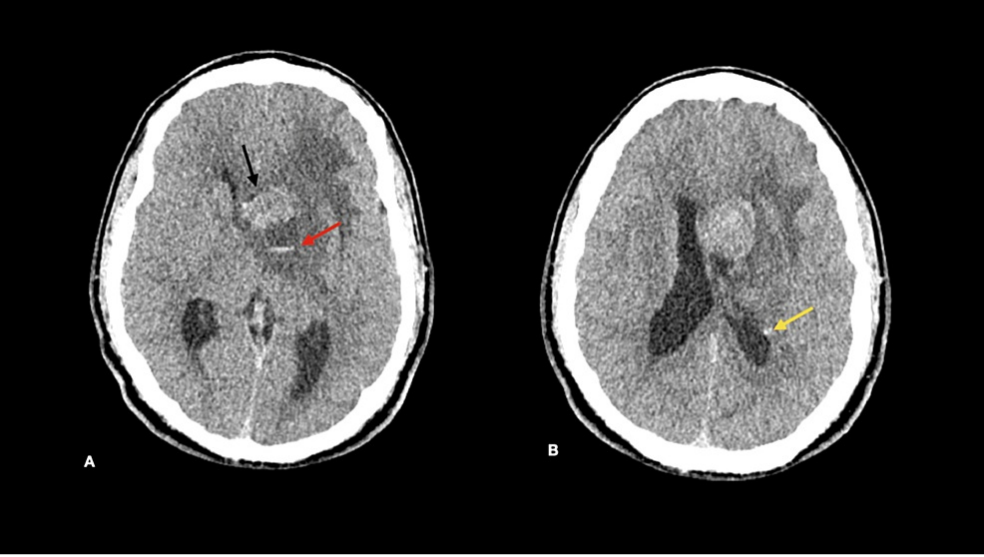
Axial unenhanced CT brain. A. showing the subependymal heterogeneously hyperdense mass (black arrow) with the cystic area showing acute blood-fluid level (red arrow). B. showing calcified subependymal nodule (yellow arrow).
